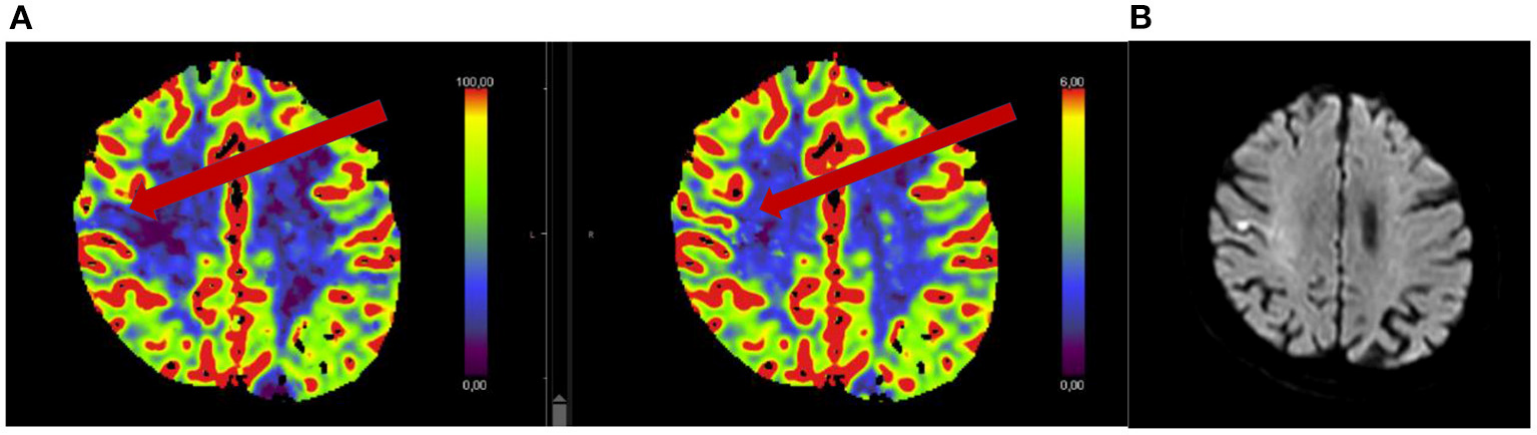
Seventy-five year old woman with acute onset of mild left hemi-body weakness and sensory loss (NIHSS 3). Patient received intravenous thrombolysis and showed clinical improvement. Acute CT perfusion showed a mismatch between normal cerebral blood volume (B) and reduced cerebral blood flow (A). Follow-up MRI performed 24 h after thrombolysis showed a small cortical ischemia.
